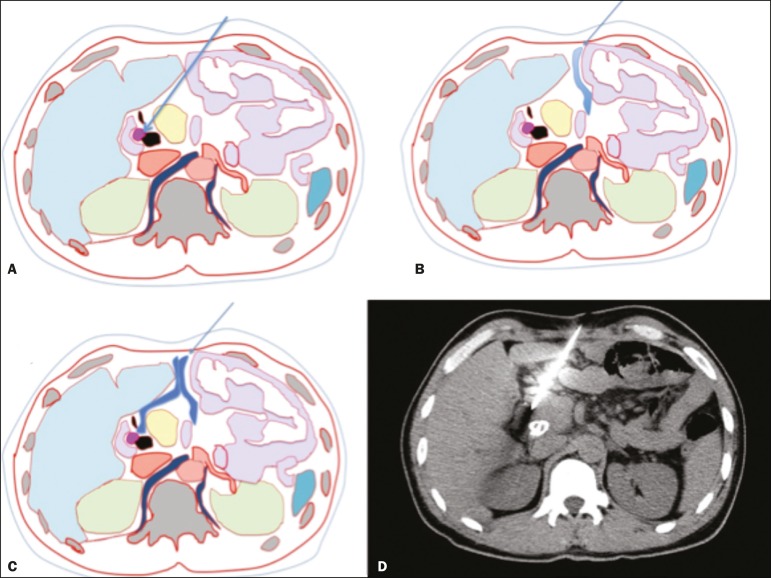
CT-guided percutaneous biopsy using the hydrodissection technique.
